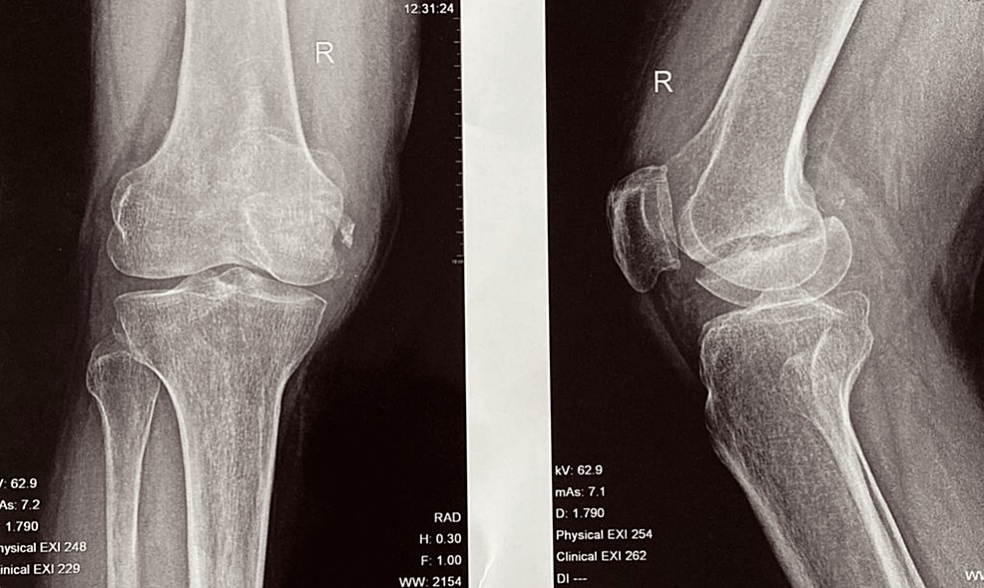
Plain radiograph of right knee in anteroposterior and lateral view showing Hoffa fracture of medial condyle
